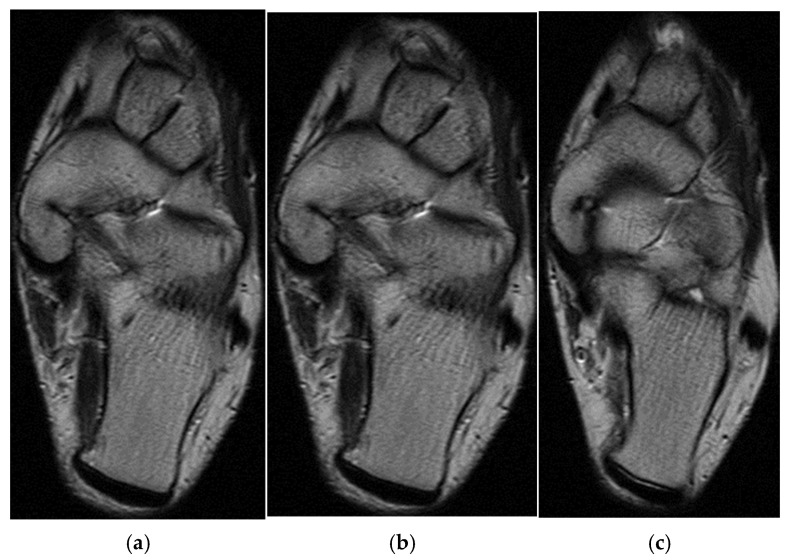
(a) Ankle MRI: axial T2 acquisition, (b) ankle MRI: axial T2 acquisition, (c) ankle MRI axial T2 acquisition.
